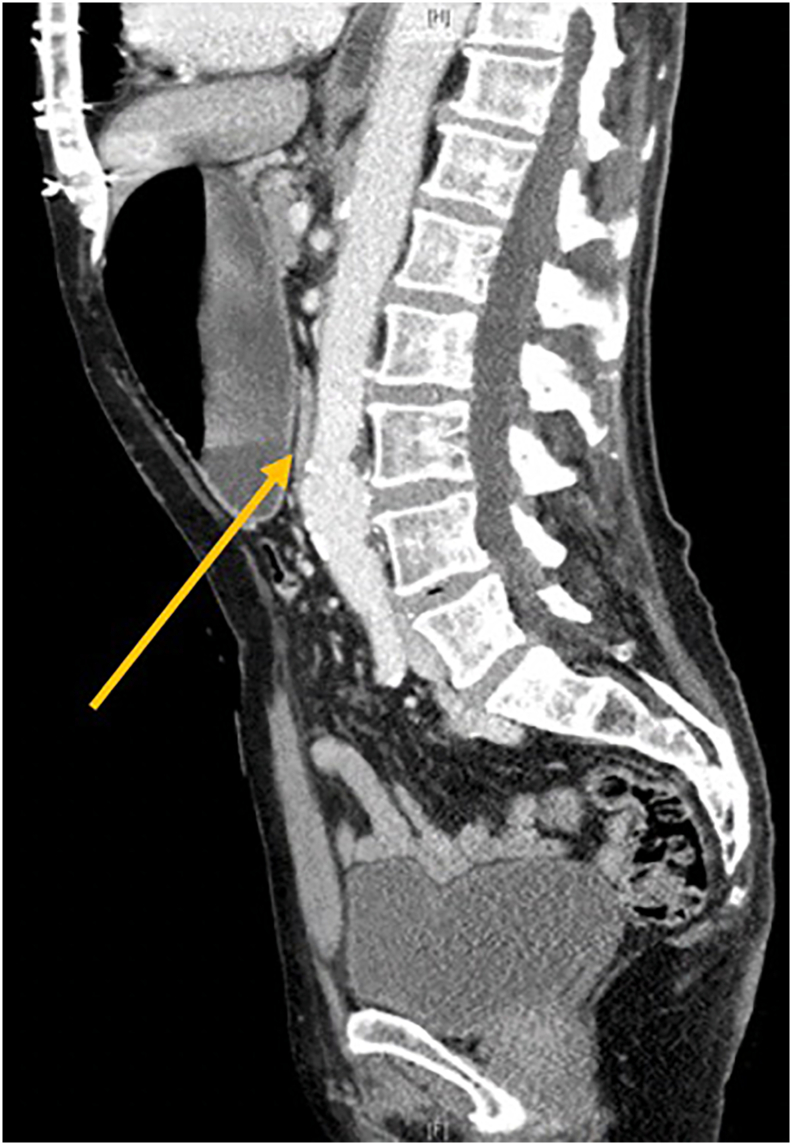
Computed tomography, sagittal view demonstrating collapsed in the 4th part of duodenum (indicated by arrow).
